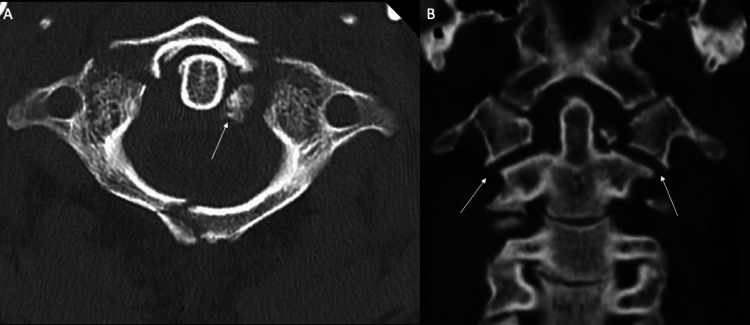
(A) Axial computed tomography shows displaced C1 fracture with transverse tubercle avulsion (white arrow). (B) Coronal images demonstrate approximately 7 mm of total lateral mass displacement (white arrows).
